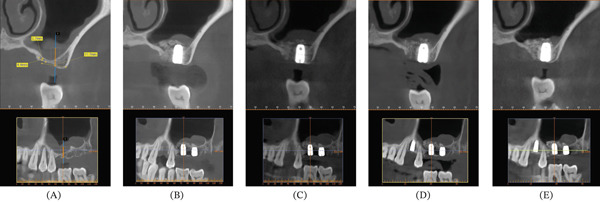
The preoperative design, postoperative, and follow‐up CBCT. (A) Preoperative design. (B) Postoperative CBCT. (C) One month postoperative CBCT. (D) Two months postoperative CBCT. (E) Seven months postoperative CBCT.
